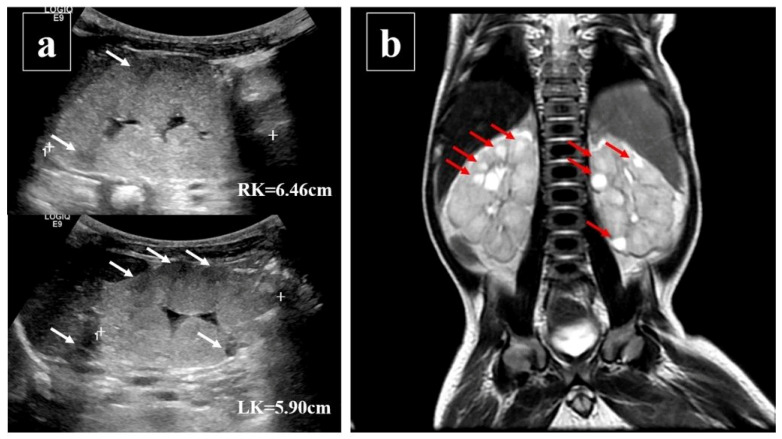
Radiological images of multicystic kidneys. (a) Renal ultrasound of multiple renal cysts (white arrows) and hyper-echoic renal parenchyma with loss of corticomedullary differentiation. (b) Renal MRI compatible with multicystic kidney disease according to the T2-weighted images (red arrows).
